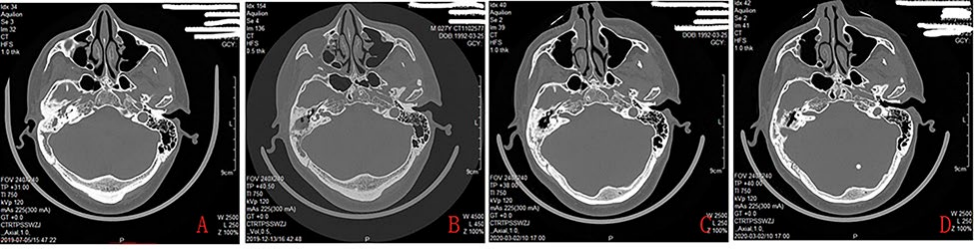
The preoperative CT revealled soft tissue density in the tympanic cavity, antrum, and aditus ad antrum (A), postoperative CT revealled well pneumatolytic middle ear (B,C, and D).Please note, this is the same patient as in Fig. 1
